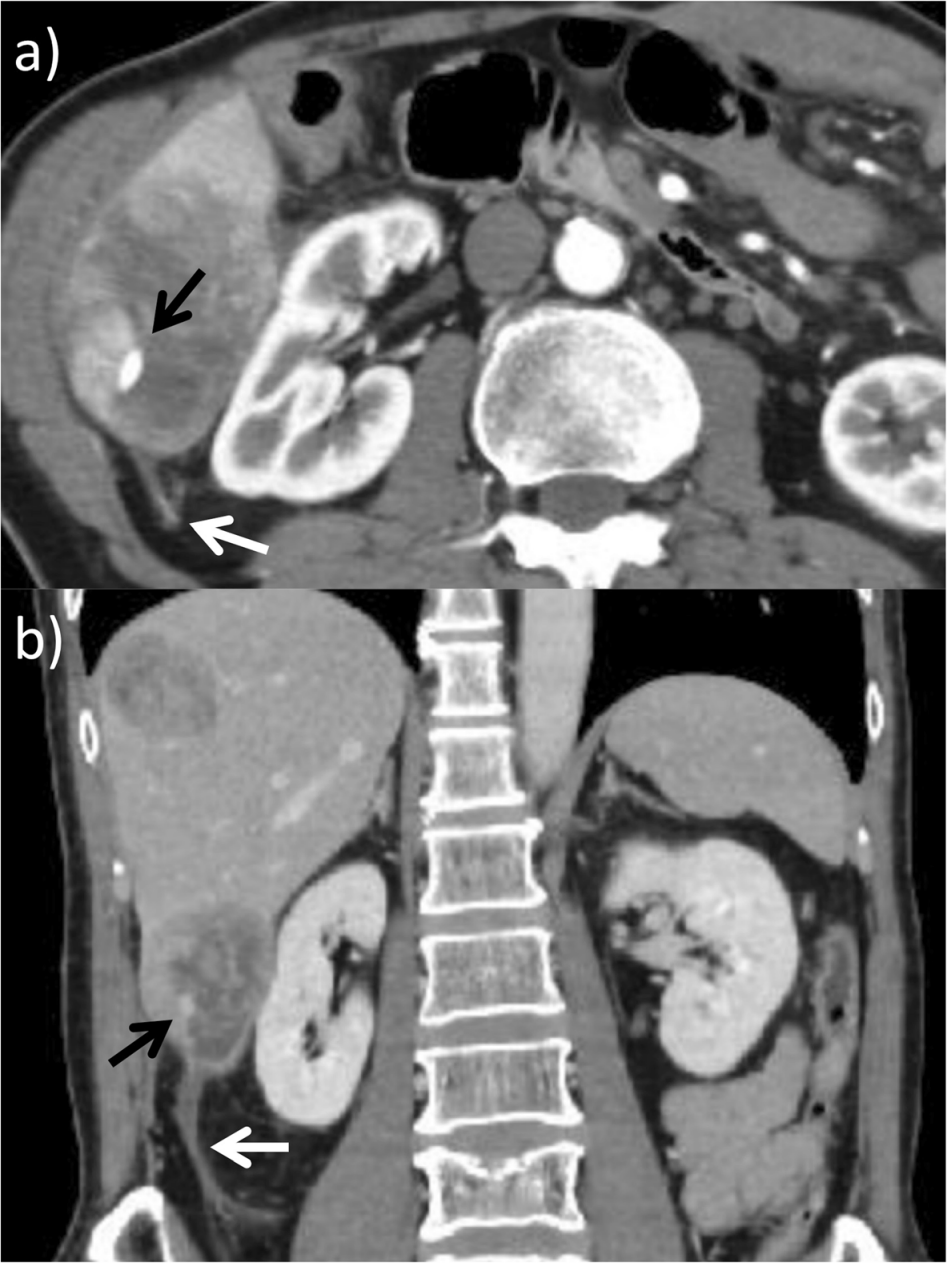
a, b CT revealed a 72 × 68 mm metastatic liver tumor in segment VI containing a 9-mm pseudoaneurysm (black arrow) and fluid collection around the hepatic edge (white arrow)
